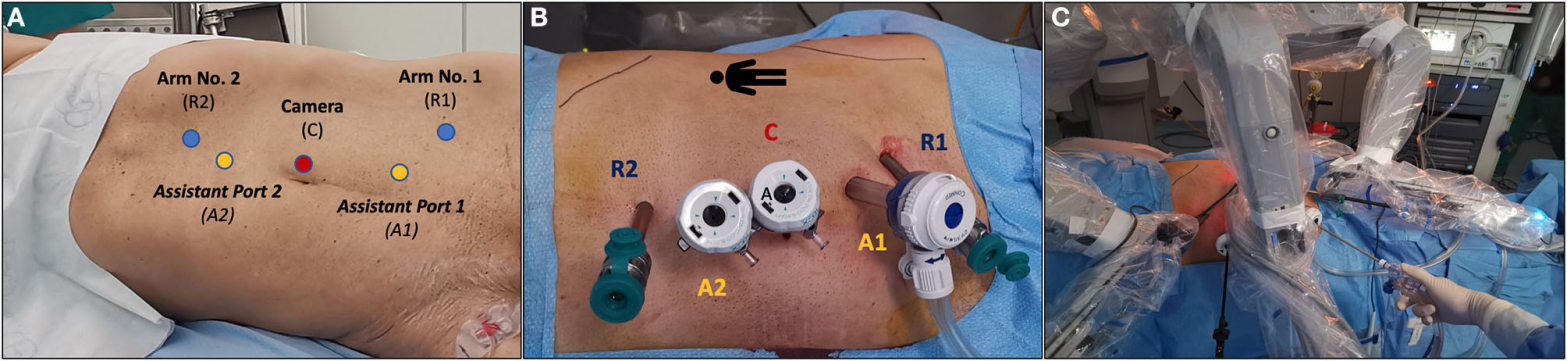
Overview of port placement in our case of robot-assisted partial nephrectomy (RAPN) for two complex renal masses in a patient with a horseshoe kidney. The da Vinci Si robotic platform with a 30° lens, a three-arm configuration plus two assistant ports [both 12-mm ports, of which one for the AirSeal® system (SurgiQuest, ConMed Corporation, Milford, CT)] was adopted. (A) Placement of the camera port using the Hasson technique plus two robotic ports and two 12-mm assistant ports. (B) Overview of the final port placement. (C) Docking of the robot.
